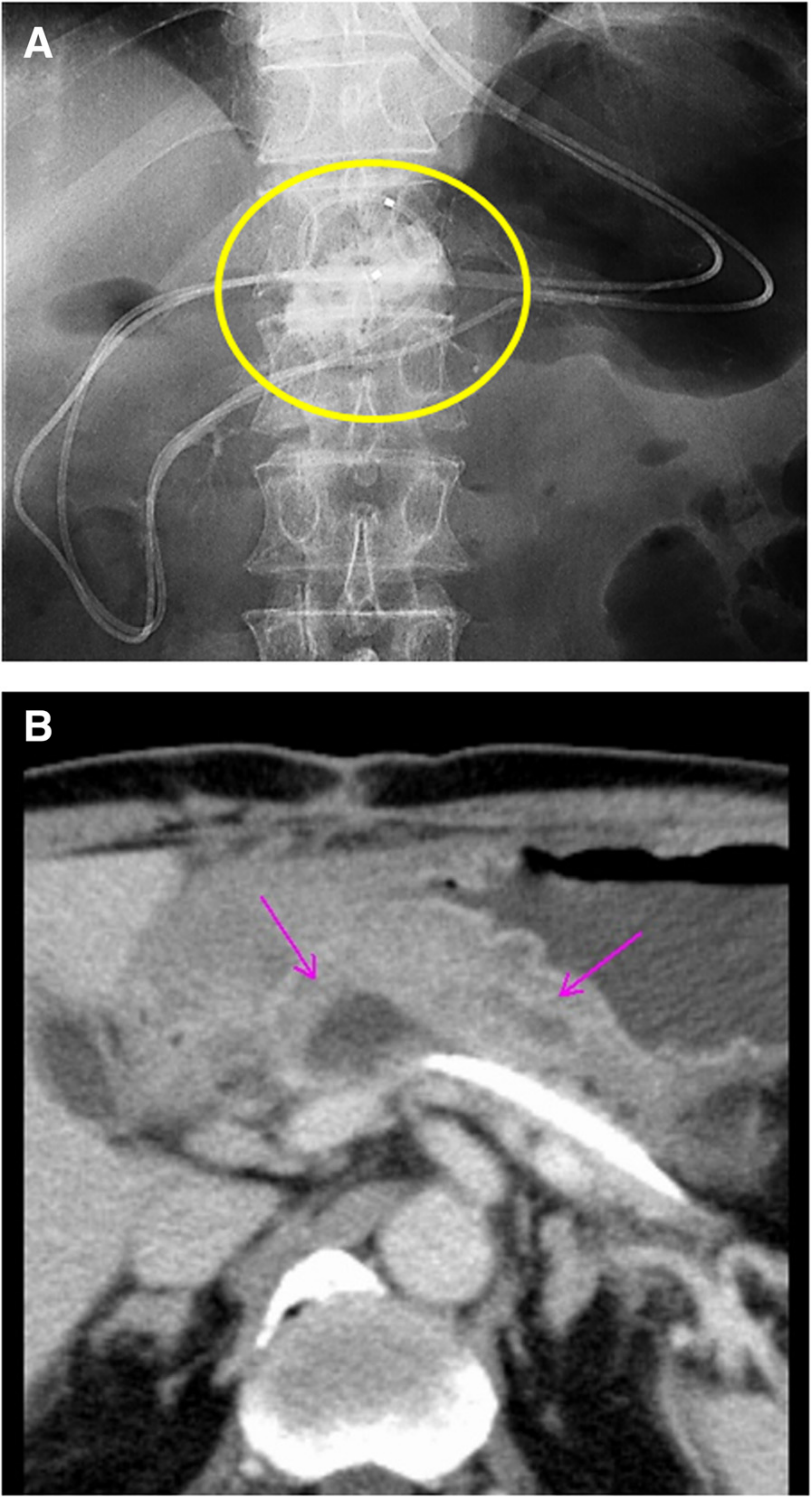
Endoscopic approach for pancreatic pseudocyst. a Two drainage tubes were inserted into the pancreatic pseudocyst and main pancreatic duct through the ampulla of Vater. b Enhanced CT showed that acute pancreatitis and pancreatic pseudocyst had recurred 1 month after endoscopic drainage
